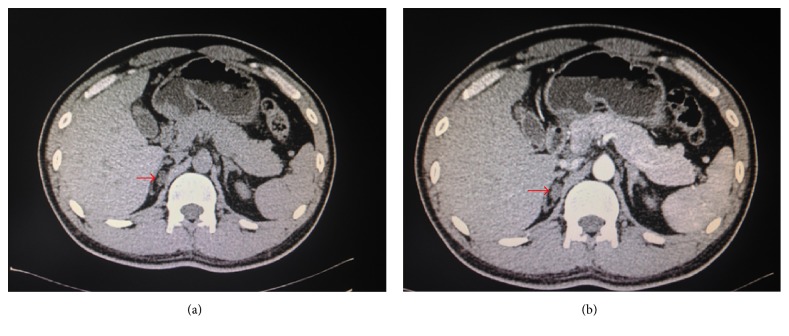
(a) Adrenal computed tomography scanning suggested right adrenal adenoma (pointed by red arrow). (b) Adrenal computed tomography enhancement indicated that the right adrenal adenoma was mildly intensified (pointed by red arrow).
